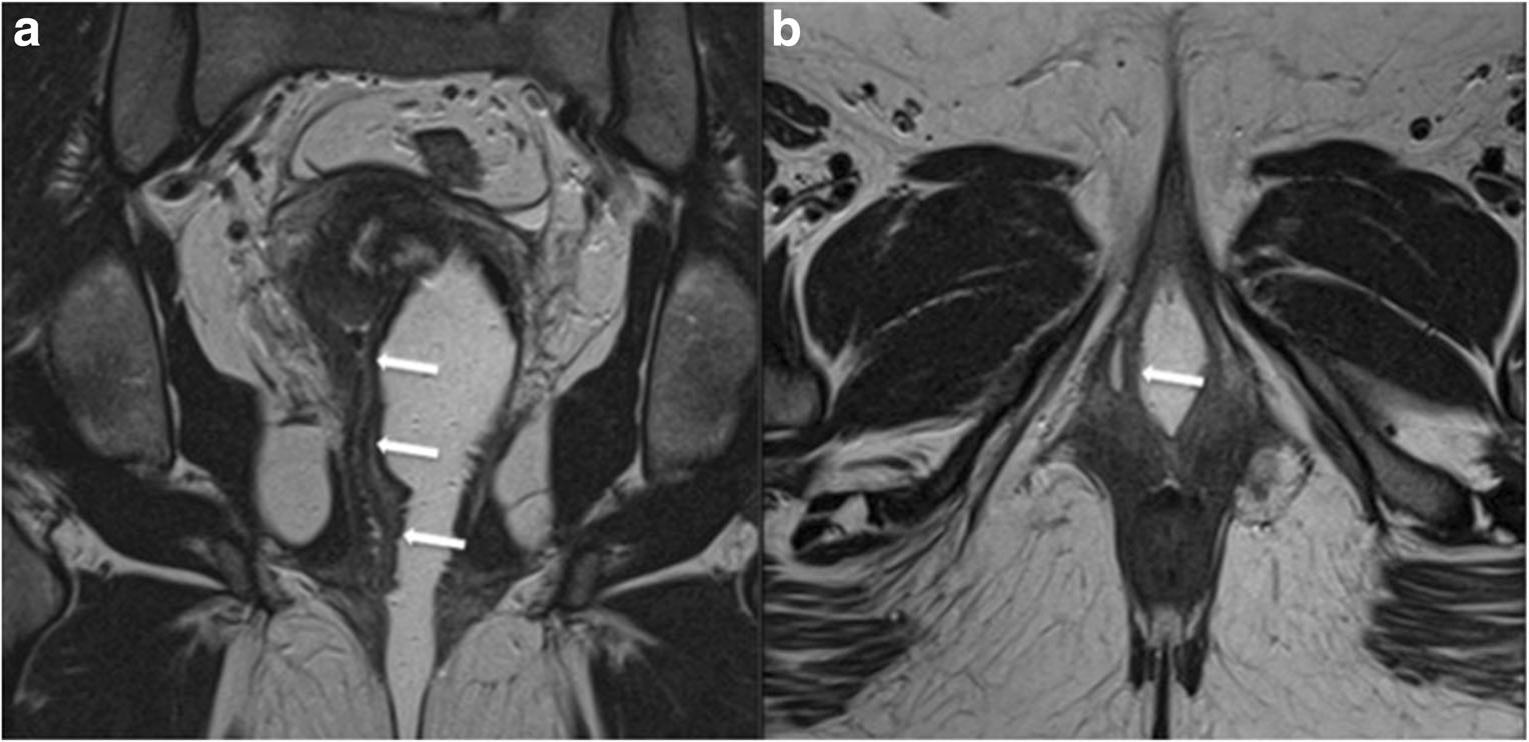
Vaginal septum revealed by MRI. T2W TSE coronal (a) and axial (b) images show a longitudinal non-obstructing vaginal septum (arrows), reaching the vaginal introitus, in keeping with a complete septum. The left vaginal canal is well distended with gel, while the right one has only a small amount, remaining collapsed. Of note, this septum was initially missed at gynaecologic examination and was unsuspected until the MRI examination
